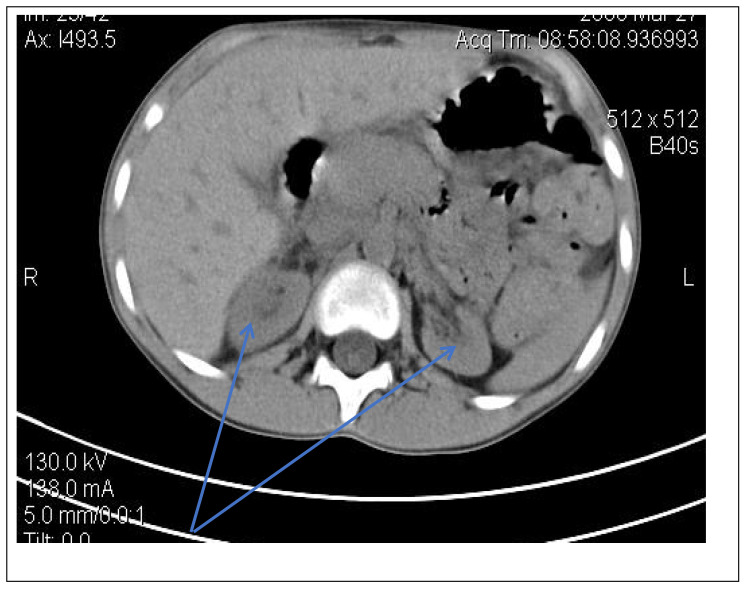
Abdominal CT scan—disappearance of renal hyperechoic formations (blue arrow).
